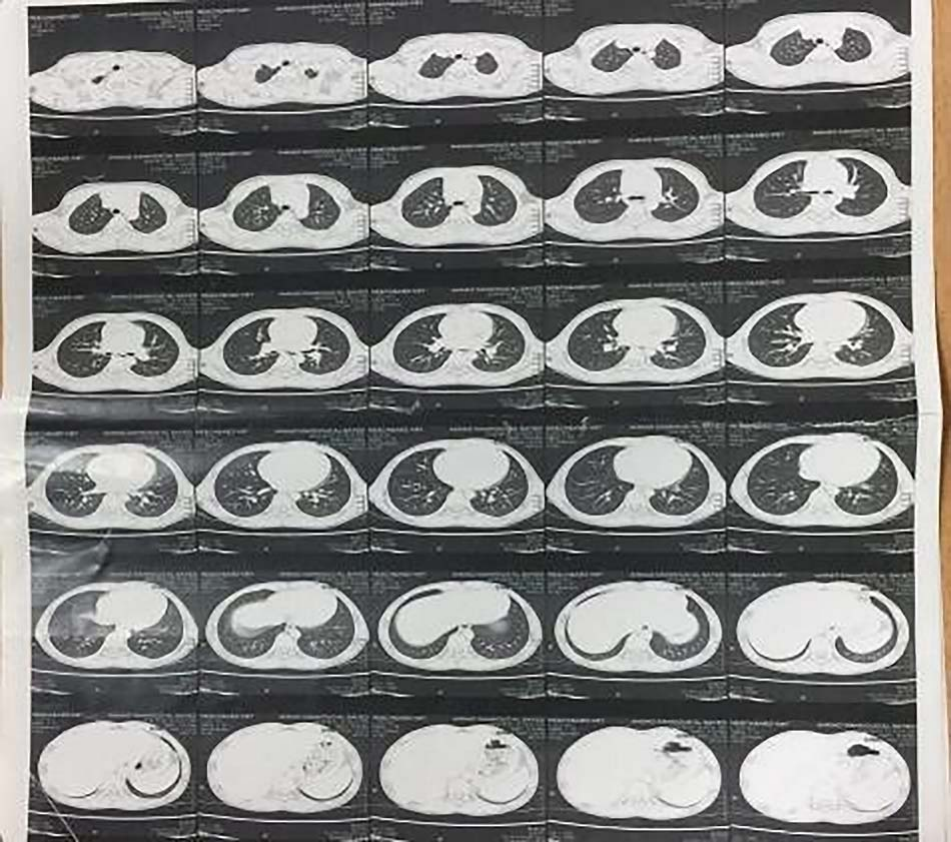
CT of slightly prominent thymus gland due to reactive hyperplasia and no significantly enlarged mediastinal lymph nodes.
